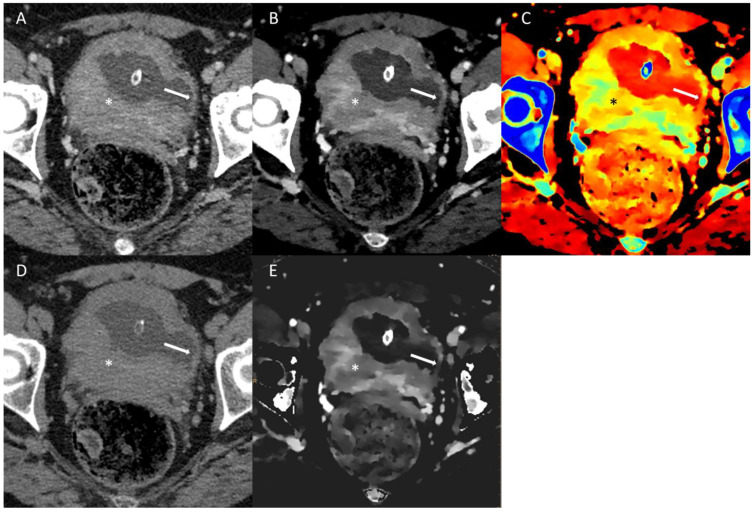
Conventional multi-energetic CT image in portal venous phase (A) of bladder cancer (*) in comparison with a small area of the healthy bladder wall (arrow). Virtual monoenergetic image (B) shows poor changes in the healthy bladder wall and inhomogeneous enhancement of the cancer. Z-effective maps image (C) shows atomic numbers within the healthy bladder wall and high numbers in cancer. Virtual Non-Contrast image (D) shows poor difference in the densitometry of the healthy bladder wall. Iodine density imaging (E) shows poor iodine concentration in the healthy bladder wall and high iodine concentration in the cancer.
